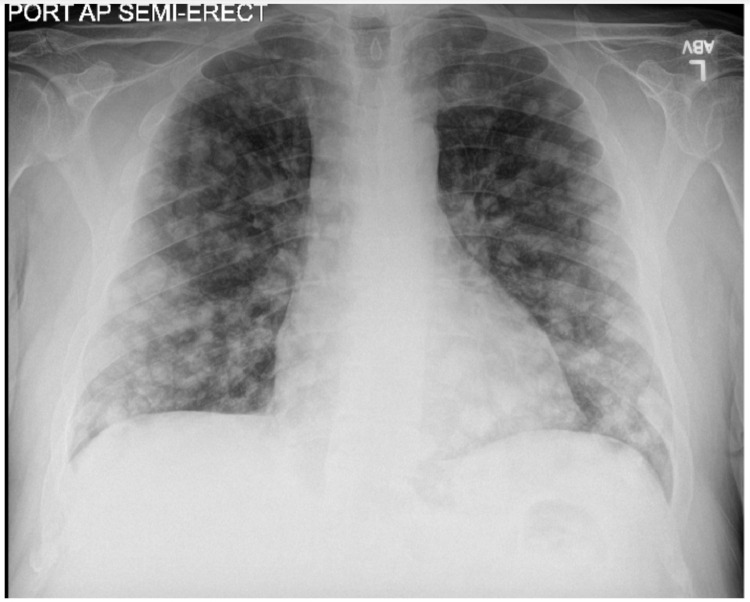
AP chest x-ray demonstrating extensive multifocal, round, nodular opacities compatible with metastatic disease.
